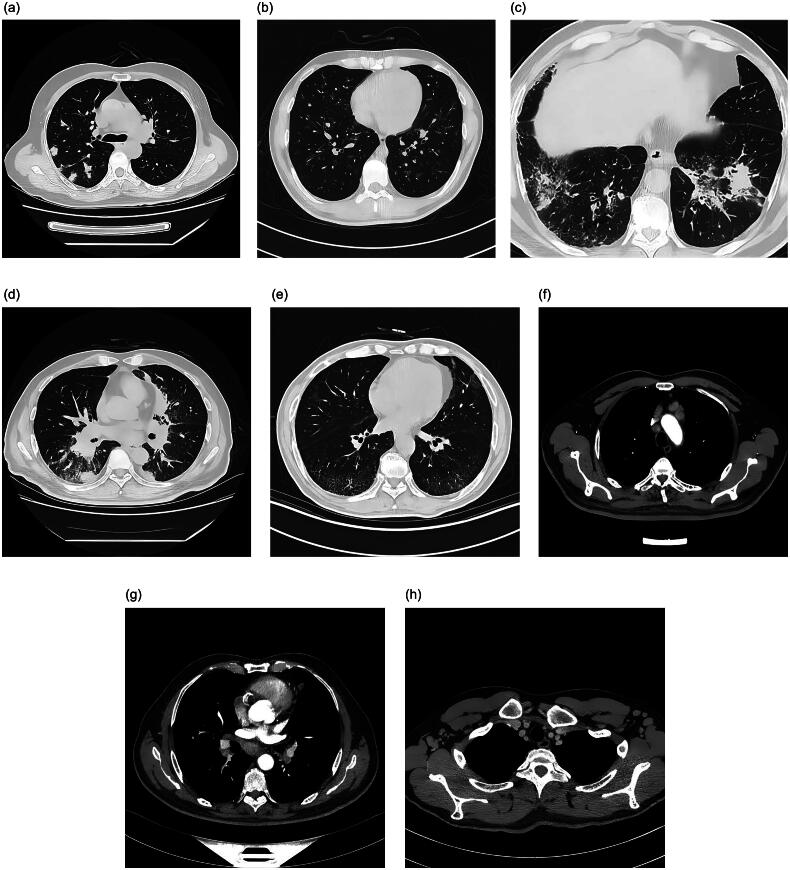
Abnormal imaging findings on chest CT. Chest abnormalities in patients with IgG4-related diseases (each computed tomography [CT] scan section is of a different patient). Axial CT scan of the lung window (a)–(e): (a) shows multiple nodules of different sizes in both lungs, (b) Thickening of bronchiolar walls in both lungs. (c) Consolidation in both lungs along bronchi. (d) Right paraphenous mass. (e) Small-grid and ground-glass opacities around the lungs, indicating interstitial changes. Mediastinal window axial enhanced CT (f)–(h): Multiple enlarged lymph nodes in the mediastinum, bilateral hila, and bilateral axillary regions.
